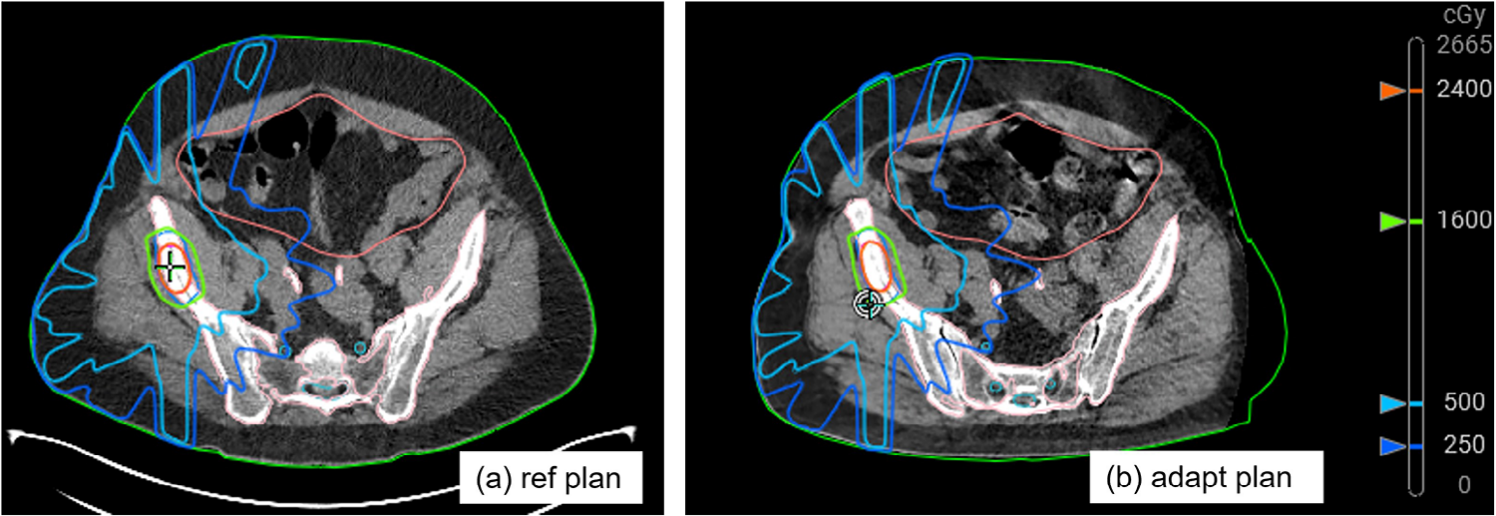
Patient reference plan on the reference diagnostic PET‐CT (a) and the adapted plan on the Ethos HS CBCT (b).
